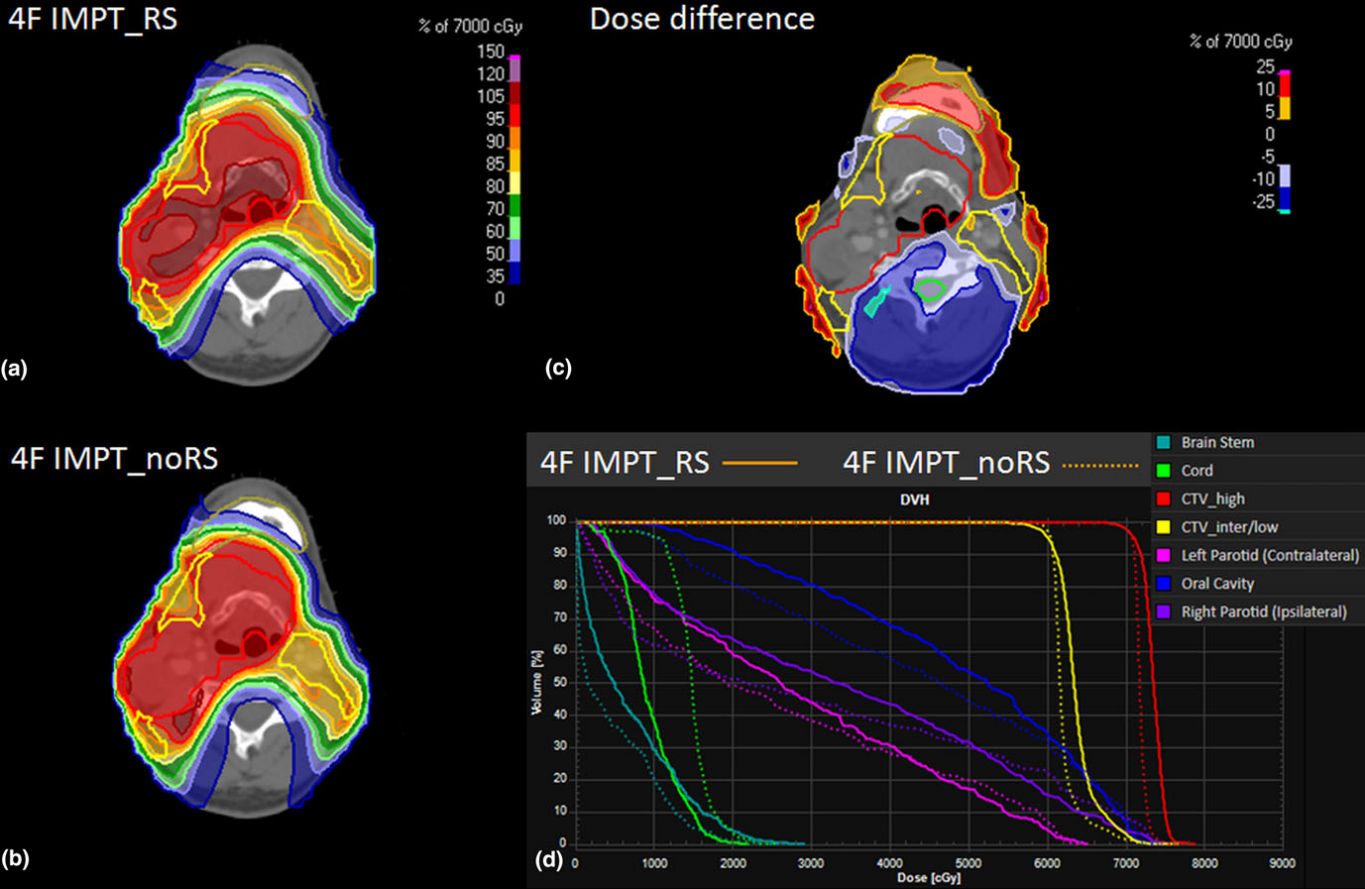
A representative CT slice and dose distribution between 4F IMPT_RS (A) and 4F IMPT_noRS (B). Dose difference: (4F IMPT_RS subtracts 4F IMPT_noRS plan); (C) and DVH's comparison (D). Both plans used the same beam angle and same objective function.
